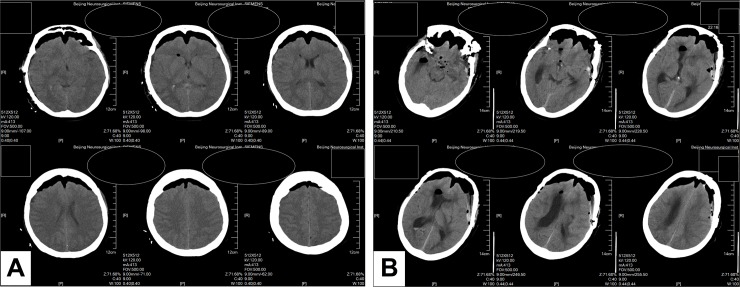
Example CT scans identified as bi-frontal pneumocephalus (A) and “Mount Fuji sign” (B). Patients name and medical record number are masked. Bi-frontal pneumocephalus (A) was defined as complying with all three: 1) subdural areas of hypoattenuation were bilaterally presented in frontal region; 2) low attenuation mass existed in at least four serial axial slices (1 cm thickness); and 3) the maximal displacement of the frontal lobe from the dura was more than 1 cm at least in one slice. “Mount Fuji sign” (B) was identified by bilateral subdural hypoattenuating collections, collapsed frontal lobes and widening of the interhemispheric space between the tips of the frontal lobes.
